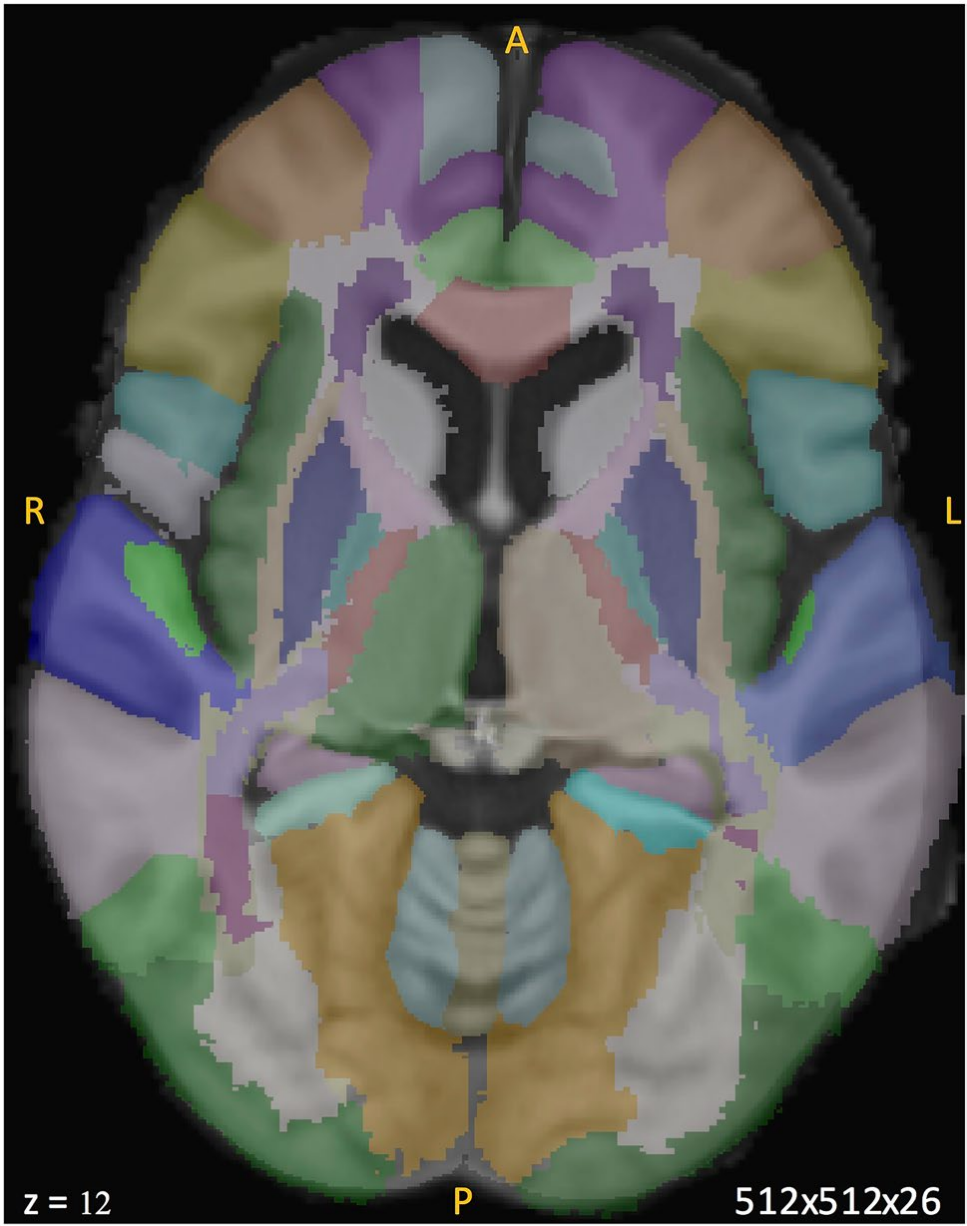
Example axial view (slice 1) of the cortical and subcortical regions of the CT-MRI atlas
